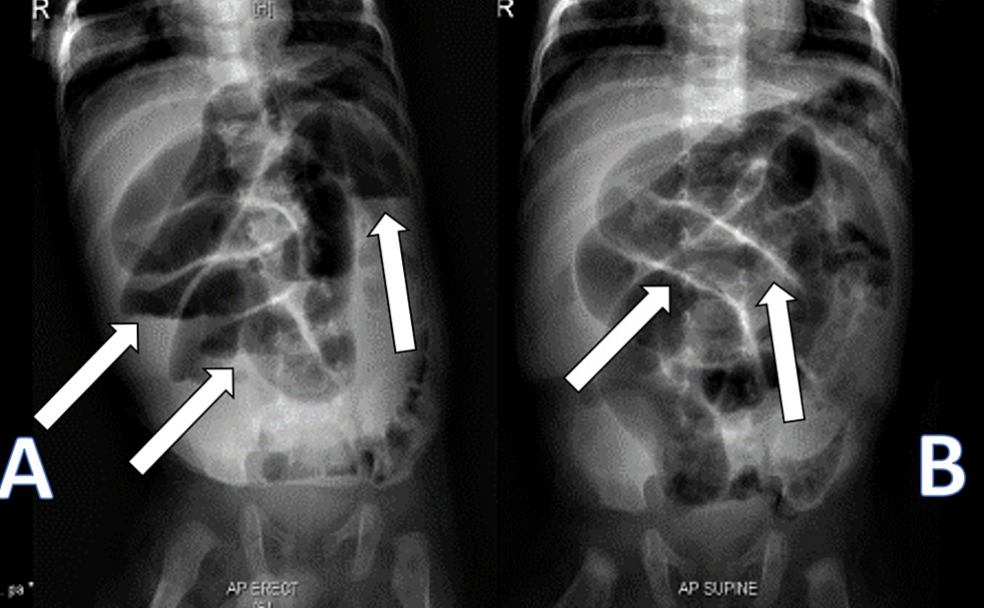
X-ray of the abdomen A: Erect X-ray of the abdomen demonstrates multiple air-fluid levels. B: Supine X-ray of the abdomen showed thickened, dilated bowel loops.
